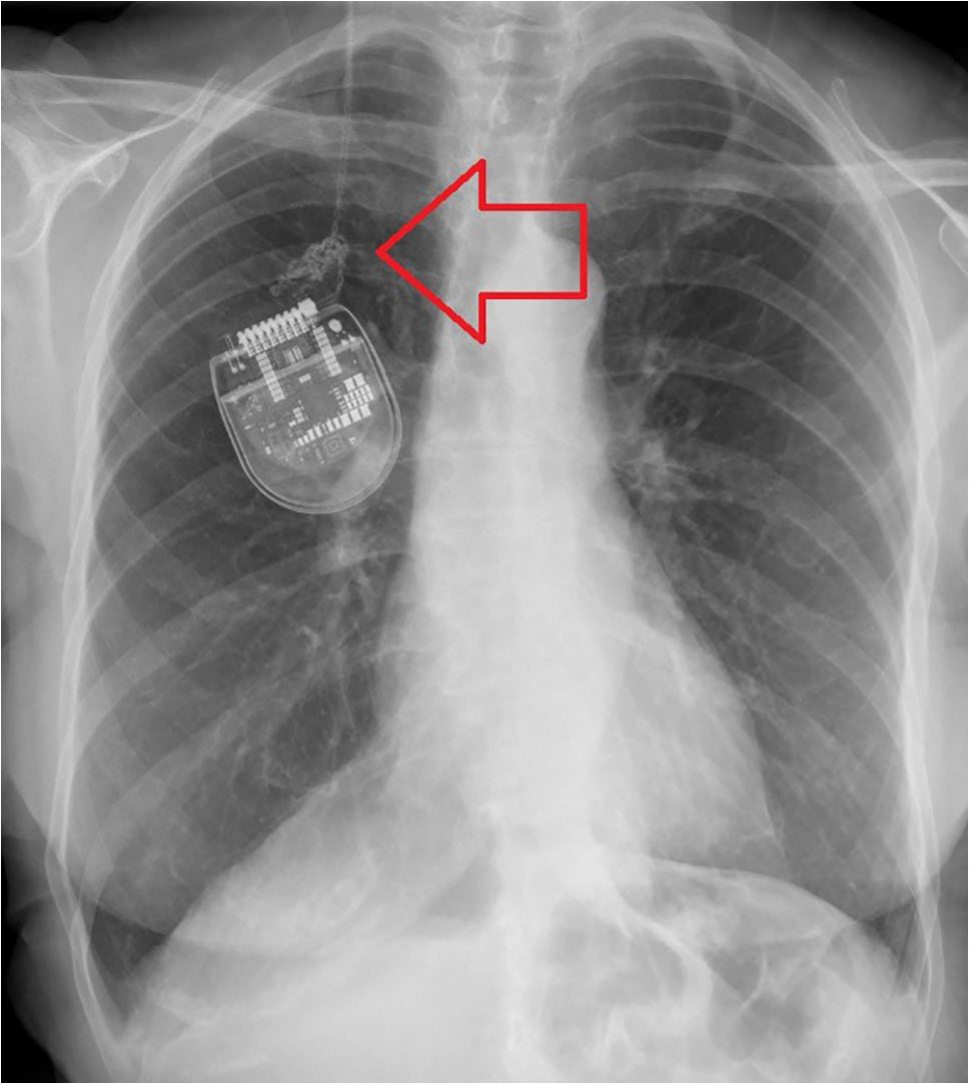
The thoracic X-ray (a.p.) shows the dislocation of the pectoral neurostimulator with twisted extensions (arrow)
